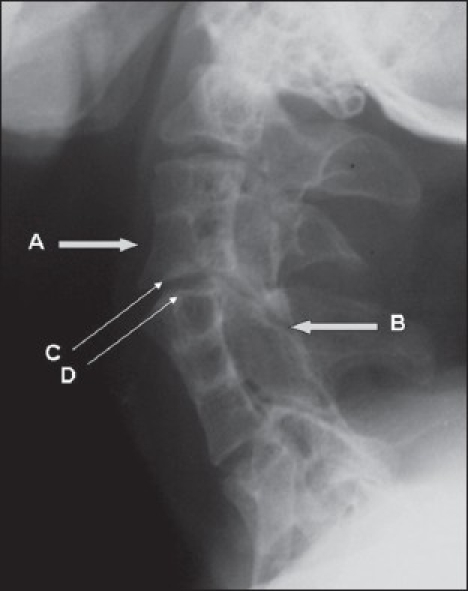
Preoperative X-ray (lateral view) cervical spine shows altered transfer of mechanical force to adjacent hypermobile segments (arrows A and B) that was further aggravated by concavo-convex joint surface (arrows C and D)
